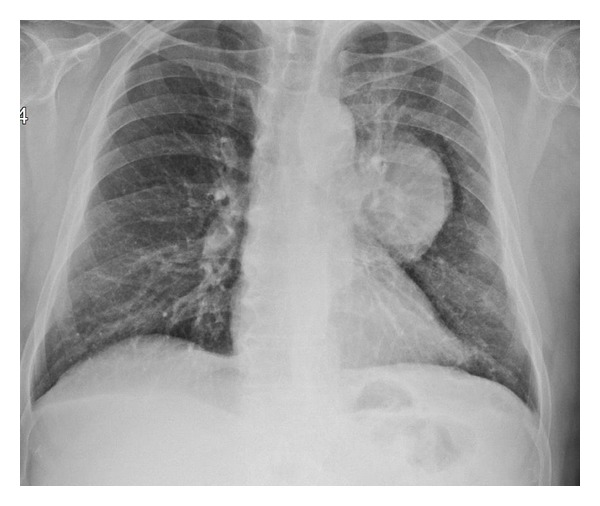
Chest X-ray of a patient showing a mass lesion located left hiler localization.
